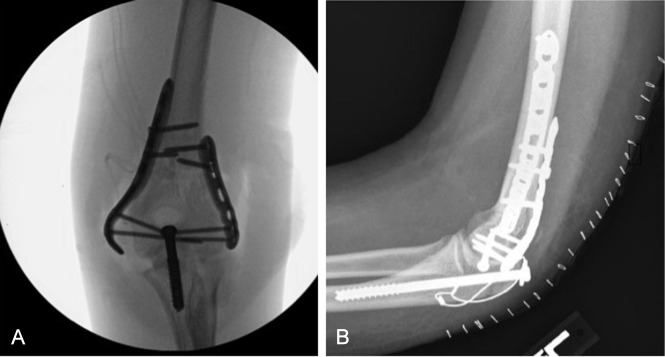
A, AP radiograph of a 13-year-old boy who fell from a ladder and sustained a T-type fracture. A large fragment screw alone was used for fixation of the olecranon osteotomy. B, Lateral radiograph in a 14-year-old girl injured in all-terrain vehicle rollover. A large fragment screw and tension band were used for fixation.
